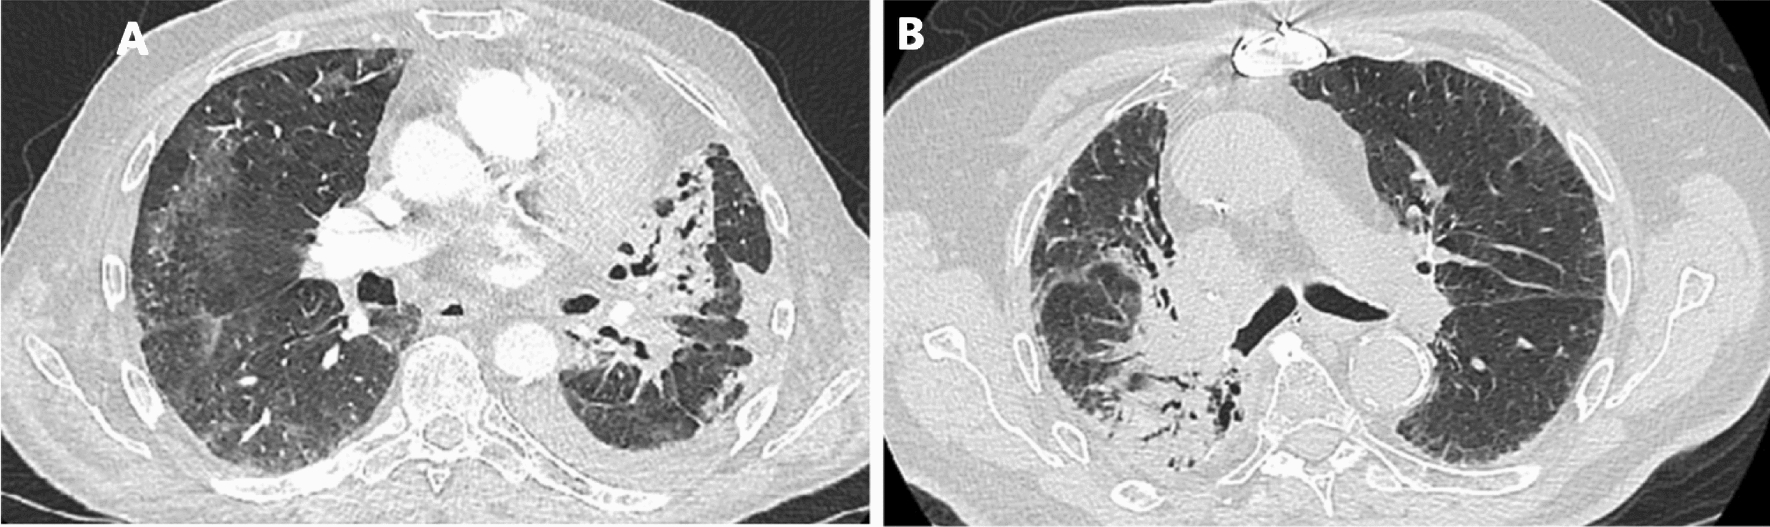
CT image of RP A: Axial image from a CT angiogram performed 5 months after radiotherapy demonstrating consolidation and atelectasis consistent with radiation pneumonitis and fibrosis. B: The image demonstrates airspace consolidation in the medial right middle lobe and superior right lower lobe with traction bronchiectasis and reticulation within the radiation field, consistent with evolution of radiation fibrosis
